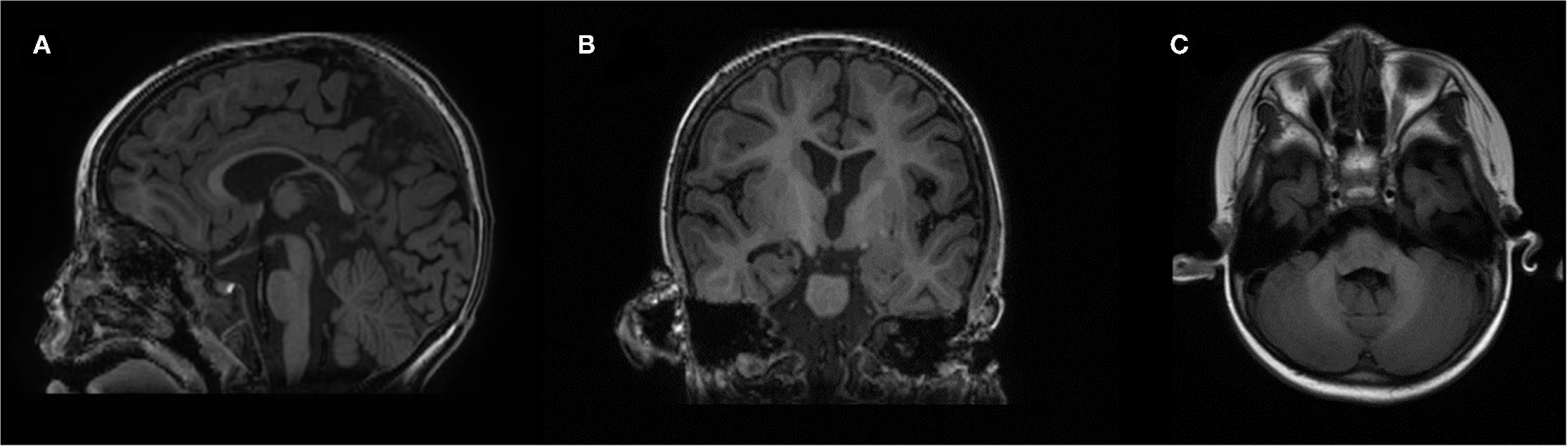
Brain magnetic resonance imaging (MRI): (A) sagittal, (B) coronal, and (C) OAx planes.
